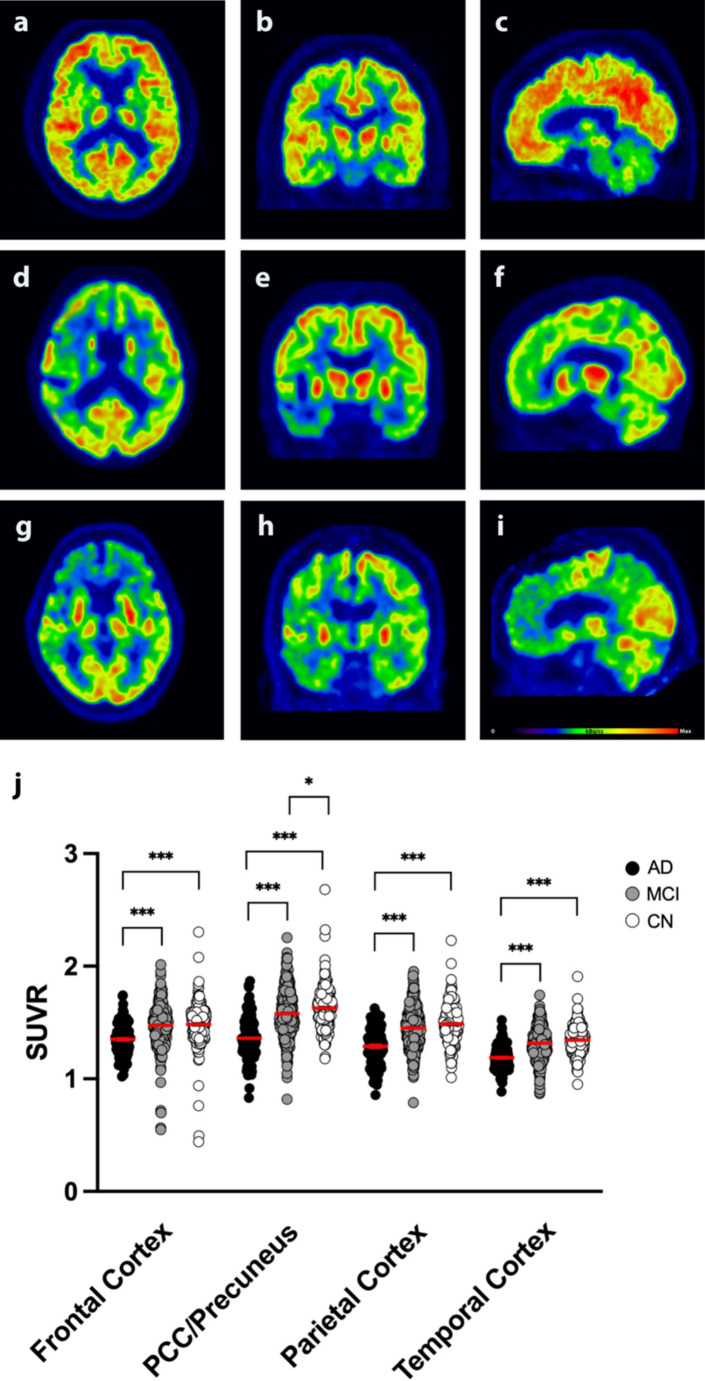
^18^F-FDG-PET. (a-c) Representative ^18^F-FDG-PET images of a cognitively normal subject in transverse (a), coronal (b), and sagittal (c) planes. (d-f) ^18^F-FDG-PET images of an MCI patient in transverse (d), coronal (e), and sagittal (f) planes. (g-h) ^18^F-FDG-PET images of an AD patient in transverse (g), coronal (h), and sagittal (i) planes. (j) SUVR was significantly lower in AD patients compared to MCI and CN patients in the frontal cortex, posterior cingulate cortex/precuneus, parietal cortex, and temporal cortex regions. ANCOVA with post-hoc Bonferroni correction; p* < 0.05; p* < 0.0001; *n* = 752. AD = Alzheimer’s dementia; MCI = Mild Cognitive Impairment; CN = Cognitive Normal; PCC = Posterior Cingulate Cortex; SUVR = Standardized Uptake Value Ratio
